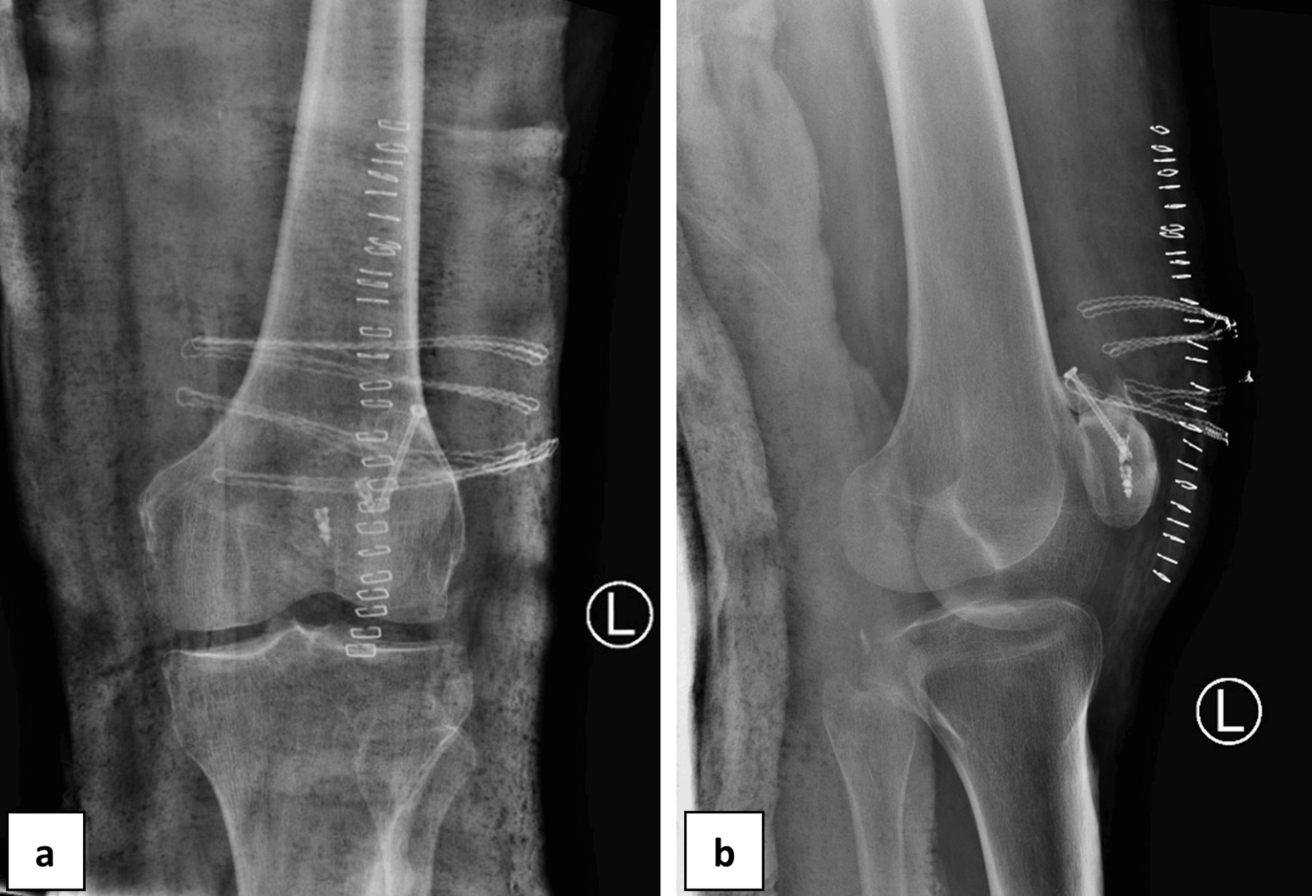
Poste-operative X-ray images Post-operative anteroposterior (a) and lateral (b) X-ray views of the left knee indicating fixation of bipartite patella separation using a canulated partially threaded screw. The quadriceps tendon was reattached using metallic bony anchors.
